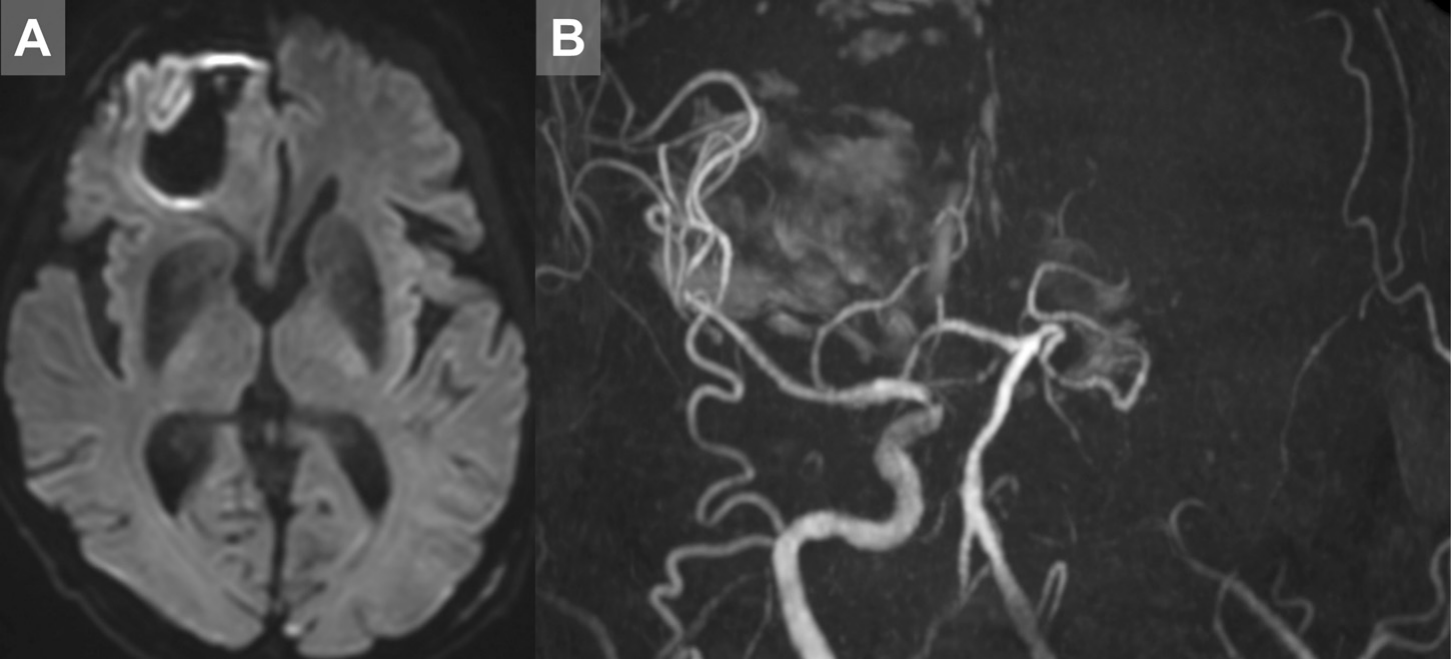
Preprocedural image (A and B) Emergency magnetic resonance imaging showing slight acute ischemic changes and occlusion of the left internal carotid artery. The bilateral anterior cerebral arteries were not visualized.
